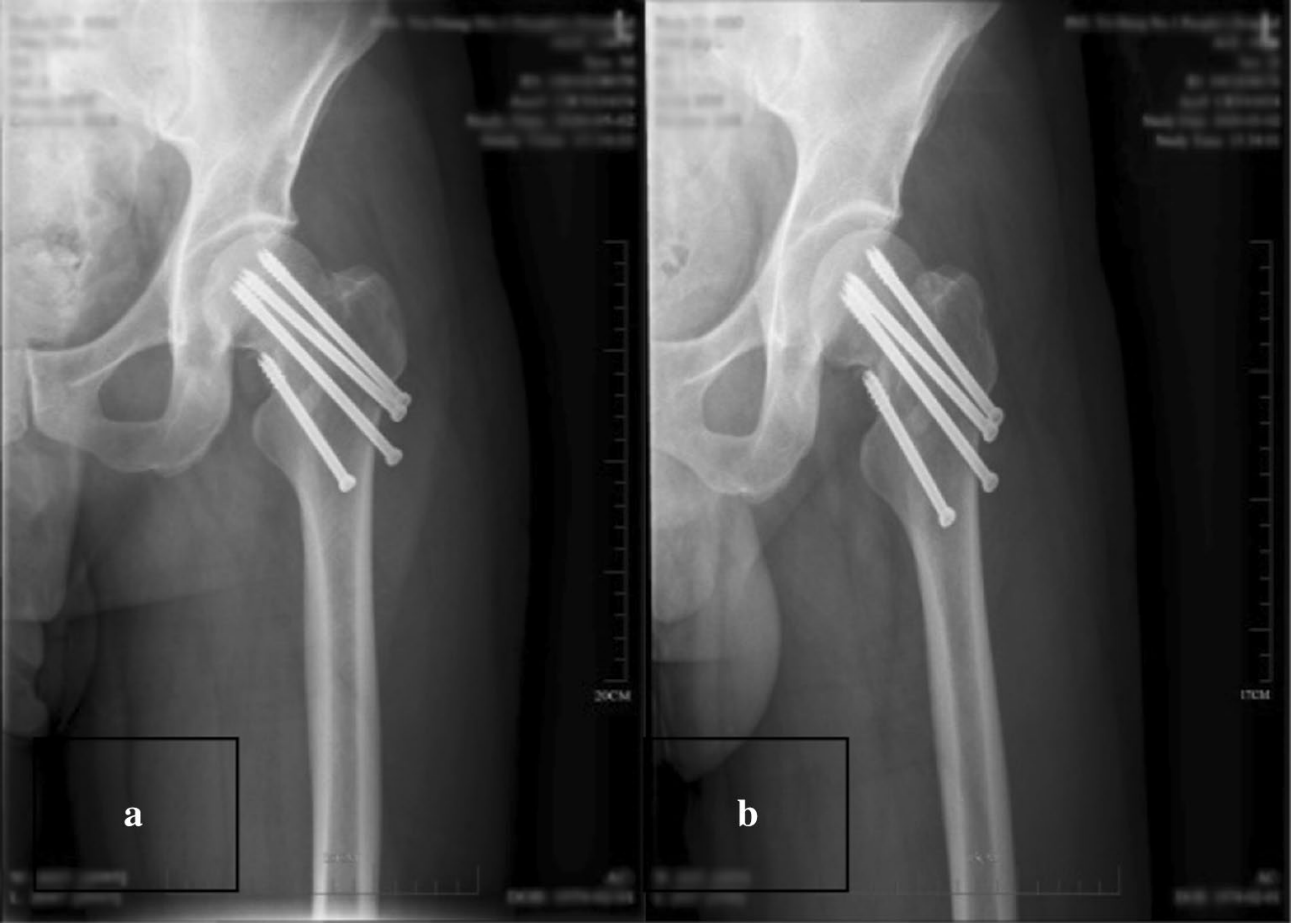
(a, b) The X-ray positive radiographs reviewed on the second day after surgery (*Note*: The patient was a 47-year-old male. He was admitted to the hospital for 2 h due to a high fall injury).
